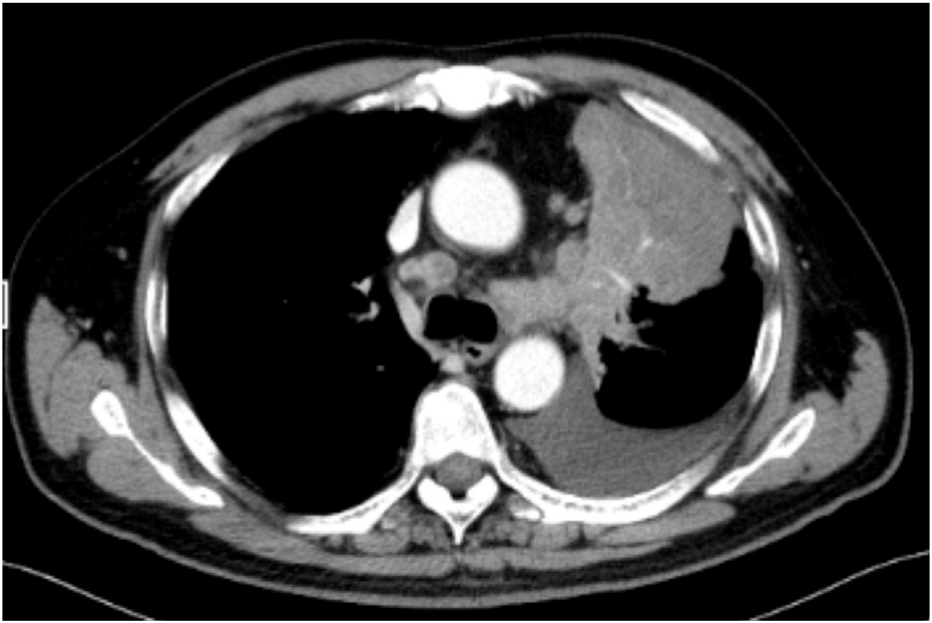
Enhanced CT scan of the chest.
